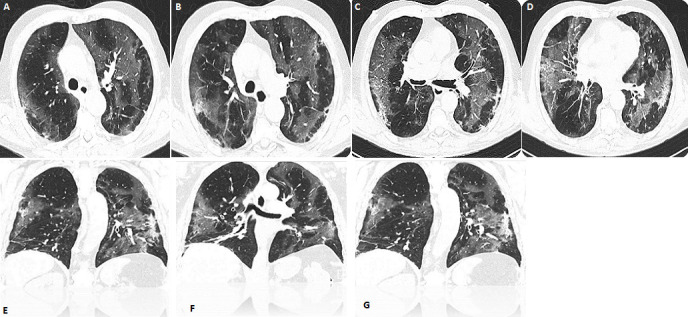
(A,B,C,D,E,F,G) CT Scan showed bilateral peripheral ground-glass opacities
